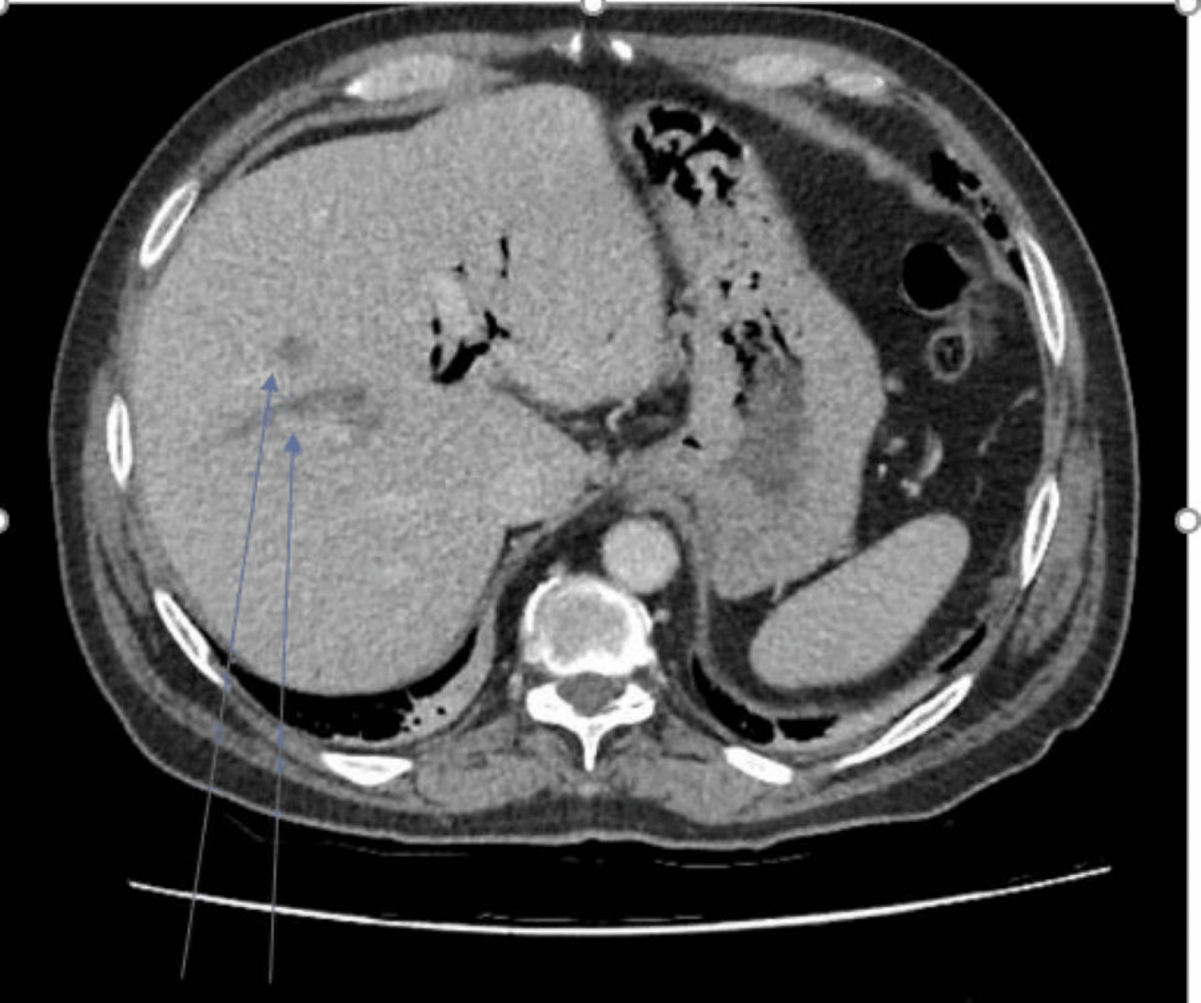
Initial CT abdomen and pelvis with hypodense liver lesions likely suggestive hepatic abcess
